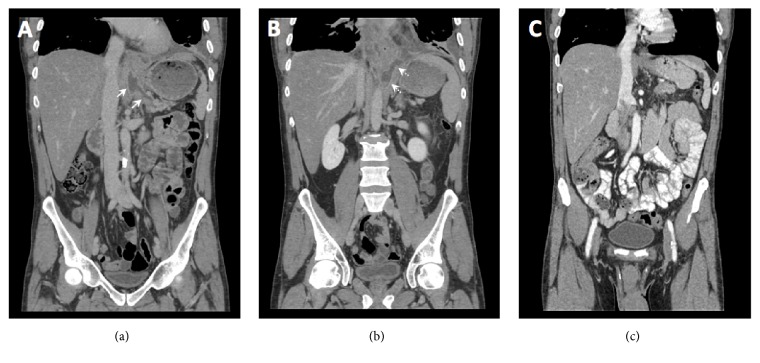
Coronal views of contrast-enhanced CT of the abdomen and pelvis show a fistula tract arising from the tail of the pancreas ((a) white arrows) extending caudally and connecting to the left pleural space ((b) dashed white arrows) with small pleural effusion and overlying atelectasis. A follow-up CT (c) demonstrates resolution of the fistula tract and pleural effusion.
